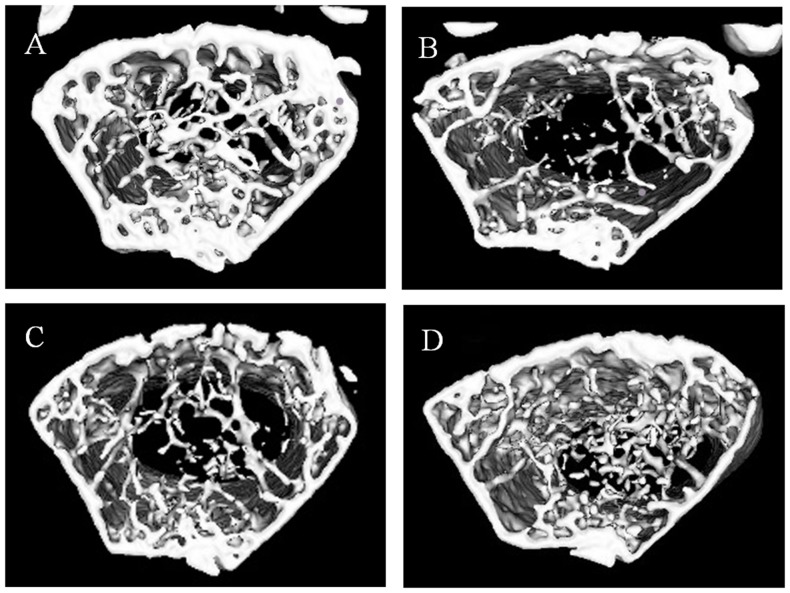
Analysis of micro-computed tomography within the distal metaphyseal femur region. A: sham, B: OVX, C: OVX+salidroside(5 mg/kg), D: OVX+salidroside(20 mg/kg).
